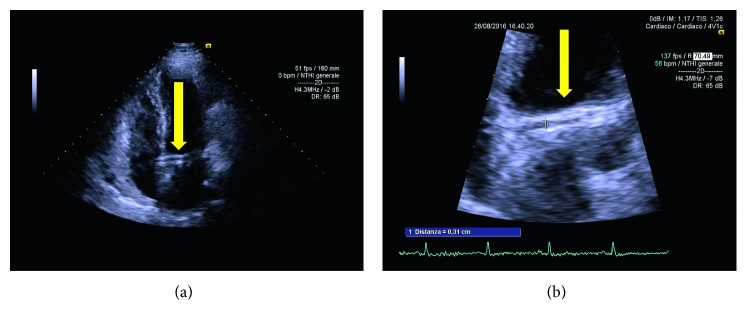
(a-b) Presentation of retroaortic course of circumflex coronary, suggesting “crossed aorta sign” in a modified apical four-chamber view behind left atrium during transthoracic study (arrows).
